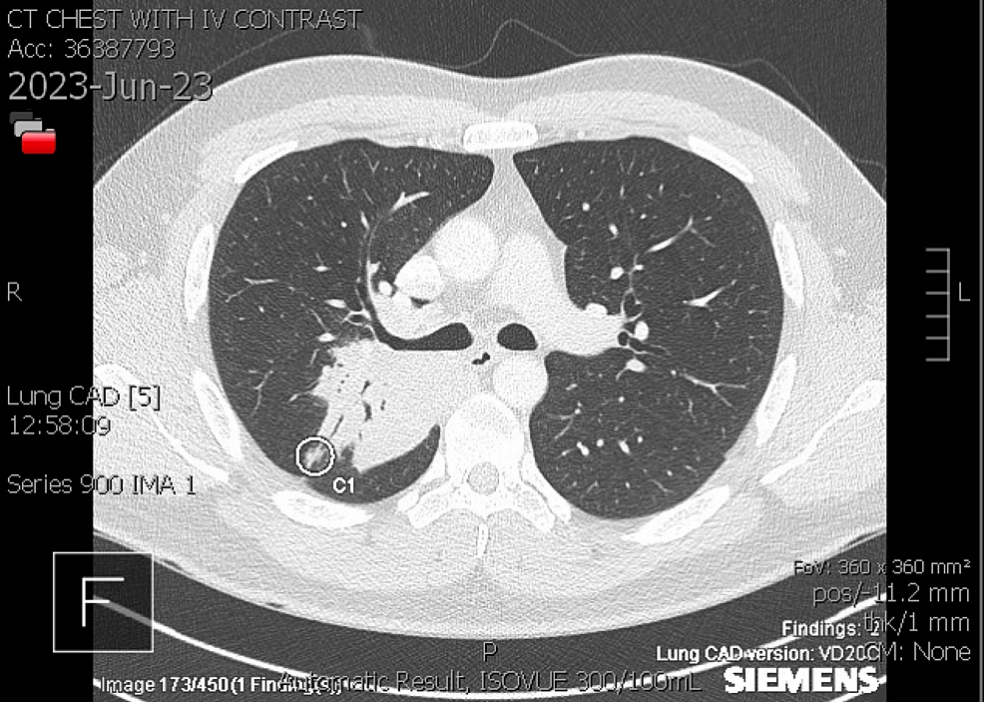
CT Scan of the chest with IV contrast depicting air bronchograms and right upper lobe posterior segment mass (axial view)
